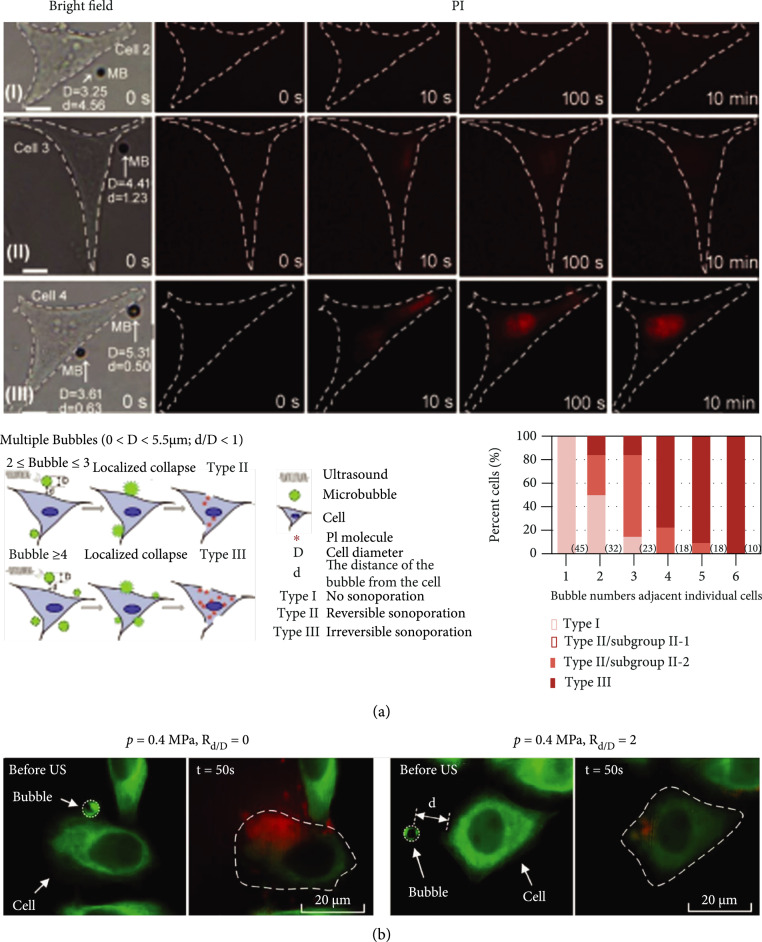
(a) The extent of sonoporation depends on bubble size and the number of bubbles adjacent to the cell. (I-III) Bright-field and propidium iodide (PI; model drug marker) fluorescence images of sonoporated cells at different times before and after a single 10 *μ*s, 1 MHz ultrasound pulse at 0.6 MPa peak negative pressure. When the bubble had a diameter (D) smaller than 5.5 *μ*m and the bubble-cell distance (d) was smaller than *D*, PI uptake (red fluorescence) shows positive correlation with bubble size and bubble number, referenced to literature [131]. (b) Real-time fluorescence observations on cellular responses induced by microbubble-mediated sonoporation at different d values. Higher PI uptake and significant cytoskeleton disassembly (green fluorescence) are observed when the ratio between d and D ($R_{d/D}$) was reduced from 2 to 0, referenced to literature [95].
